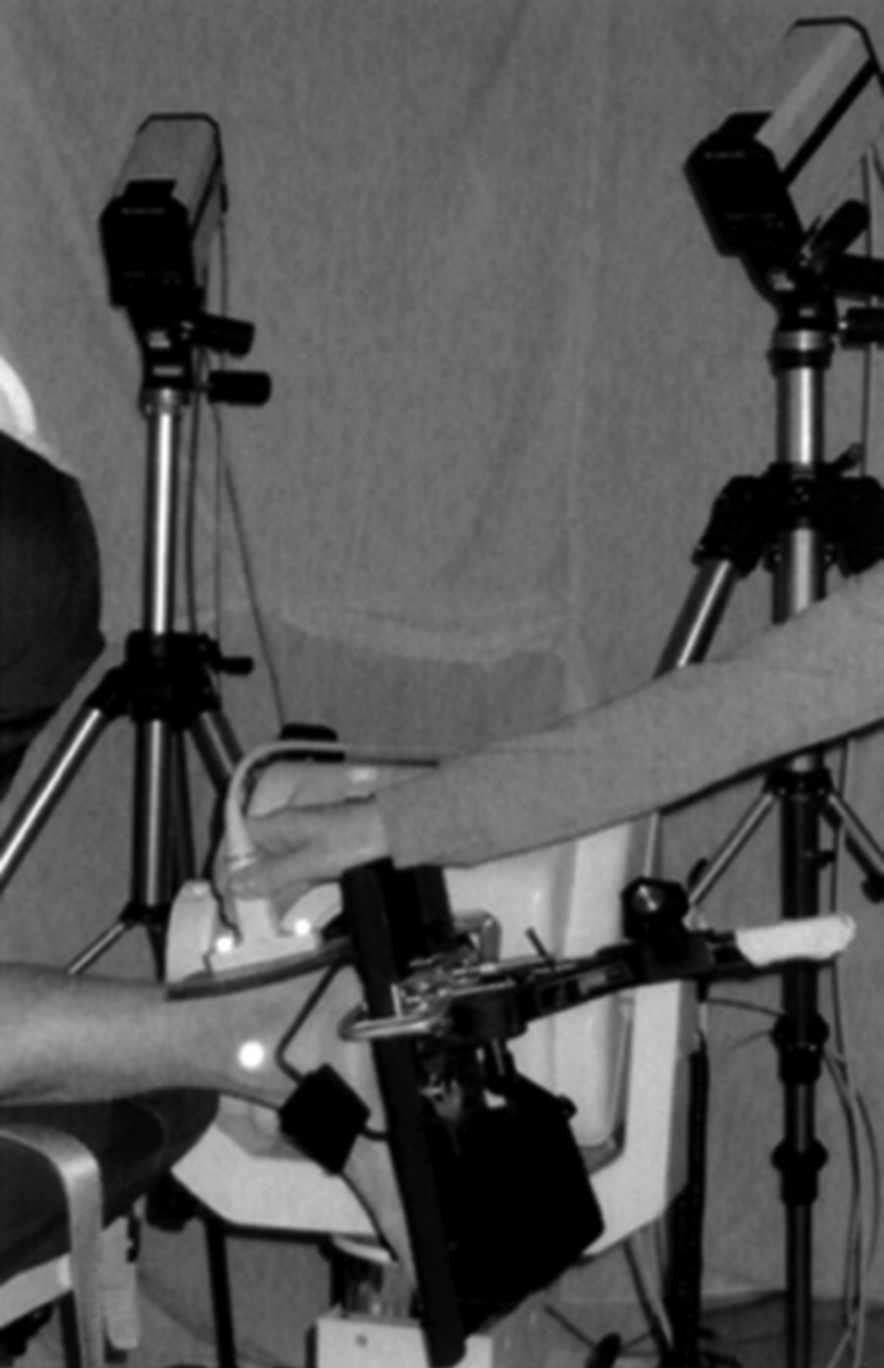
Subjects faced the back of the Biodex chair with the foot securely fastened to the foot plate. The ultrasound probe was positioned directly over the Achilles tendon and between the markers over the malleoli. Note the retroreflective markers on the casing of the ultrasound probe and use of gel pad during imaging.
